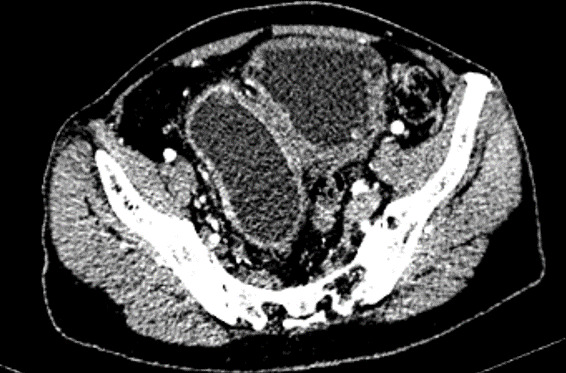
CT Scan showing a polypoidal enhancing mass arising from the bladder diverticulum.
